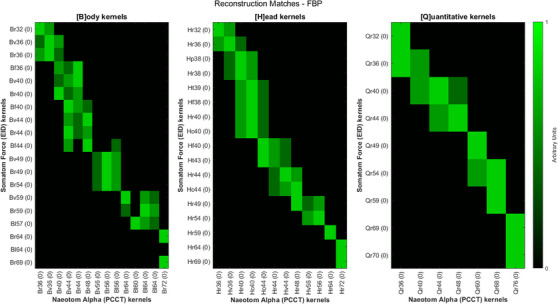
Final reconstruction match values for all kernels with filtered back‐projection (FBP) reconstruction. Matches for IR and FBP can be found in supplemental Figures. A value of 1 indicates that a particular PCCT kernel/IR combination is the best possible match for a given EID kernel/IR combination.EID, energy integrating detectors; FBP, filtered back‐projection; IR, Iterative reconstruction; PCCT, Photon‐counting computed tomography.
